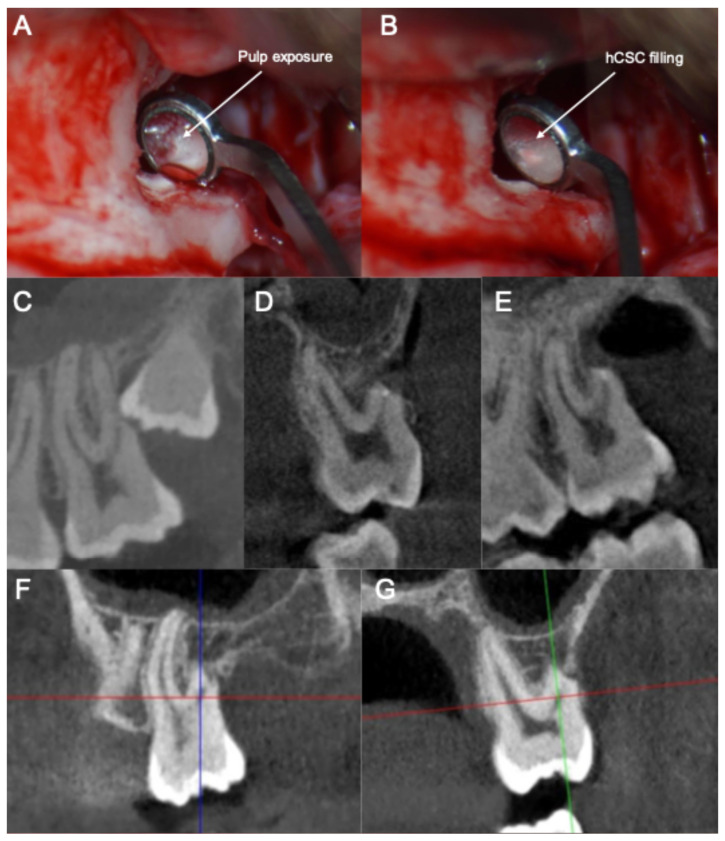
Clinical and radiographic documentation of the retrograde vital pulp treatment of the distal root of the upper left second molar due to the impaction of the upper left third molar. (A) Trimmed surface of the distal root of the second molar after the extraction of the third molar. (B) Retrograde pulp capping with a calcium silicate-based cement. (C) Impacted third molar and the resorption of the distal root of the second molar (preoperatory). (D,E) Post-operatory three-dimensional sealing of the pulp exposure (sagittal view (D); coronal view (E)). (F,G) Presence of osseous tissue neoformation surrounding the distal root of the second molar after 2 years of follow-up (coronal view (F); sagittal view (G)).
